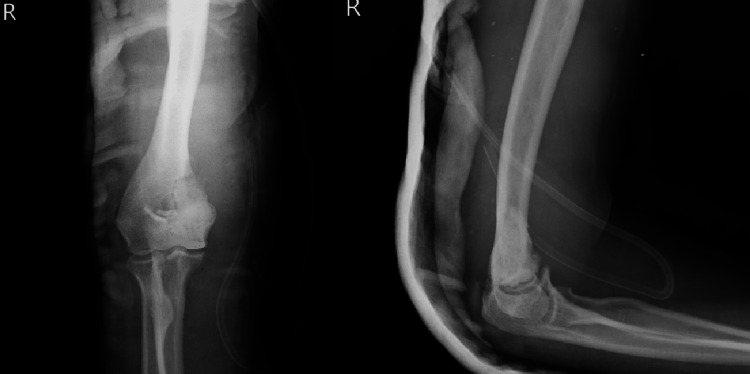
Immediate post-operative X-ray Anteroposterior and lateral views of the right elbow
